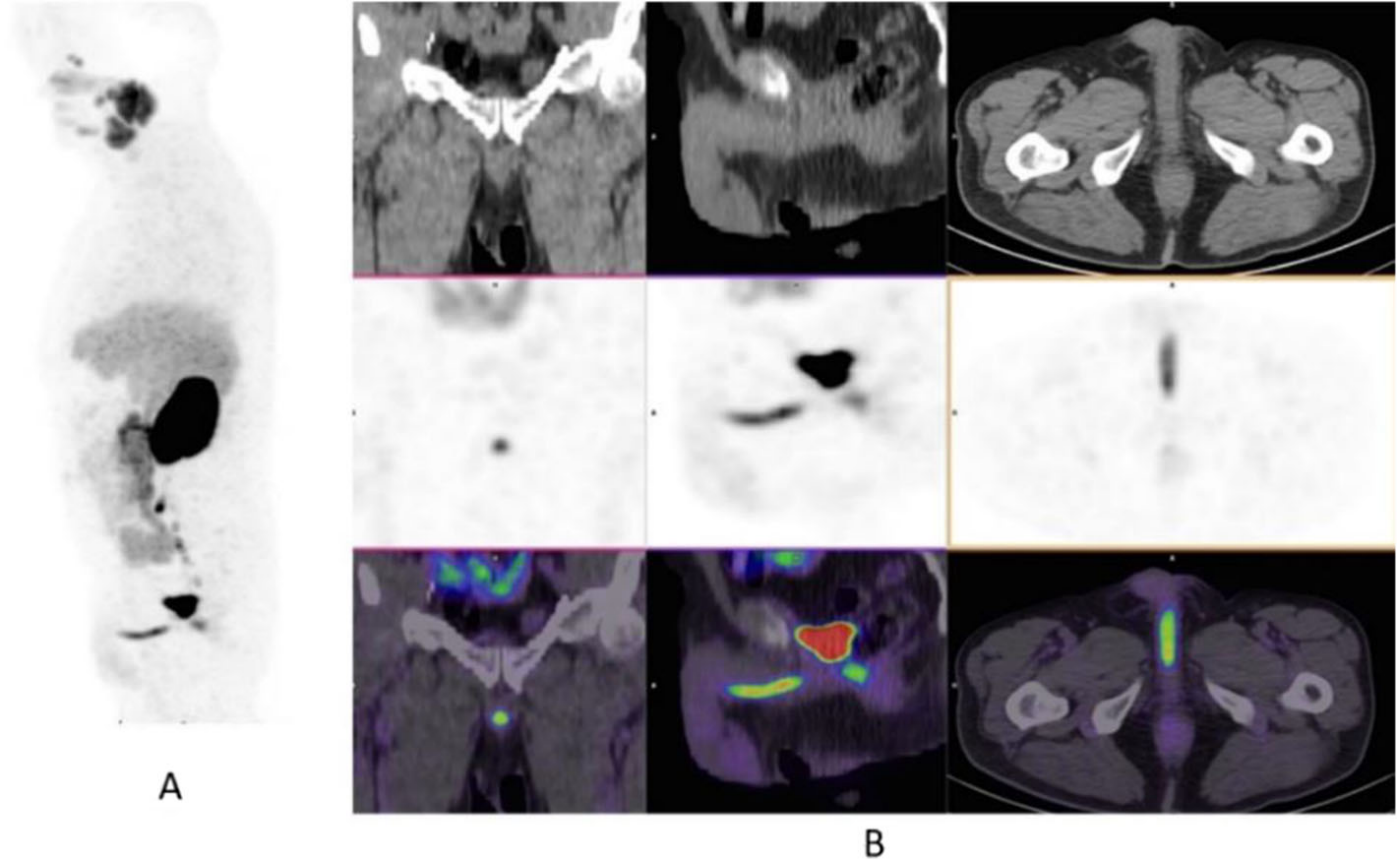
^68^Ga-PSMA, prostate cancer, biochemical recurrence (BCR): specificity. *Clinical history*: 68 y.o. man with prostate cancer, prostatectomy as primary treatment, Gleason score 4 + 5; pT3N0Mx + adjuvant radiotherapy. Three years later: PSA = 2.49 ng/ml, PSAdt 8 months. *PET/CT findings*: no evidence of recurrent disease, but urinary activity is evident in the prostatic fossa and the urethra. a MIP in a lateral view; b CT, PET, and fused images
